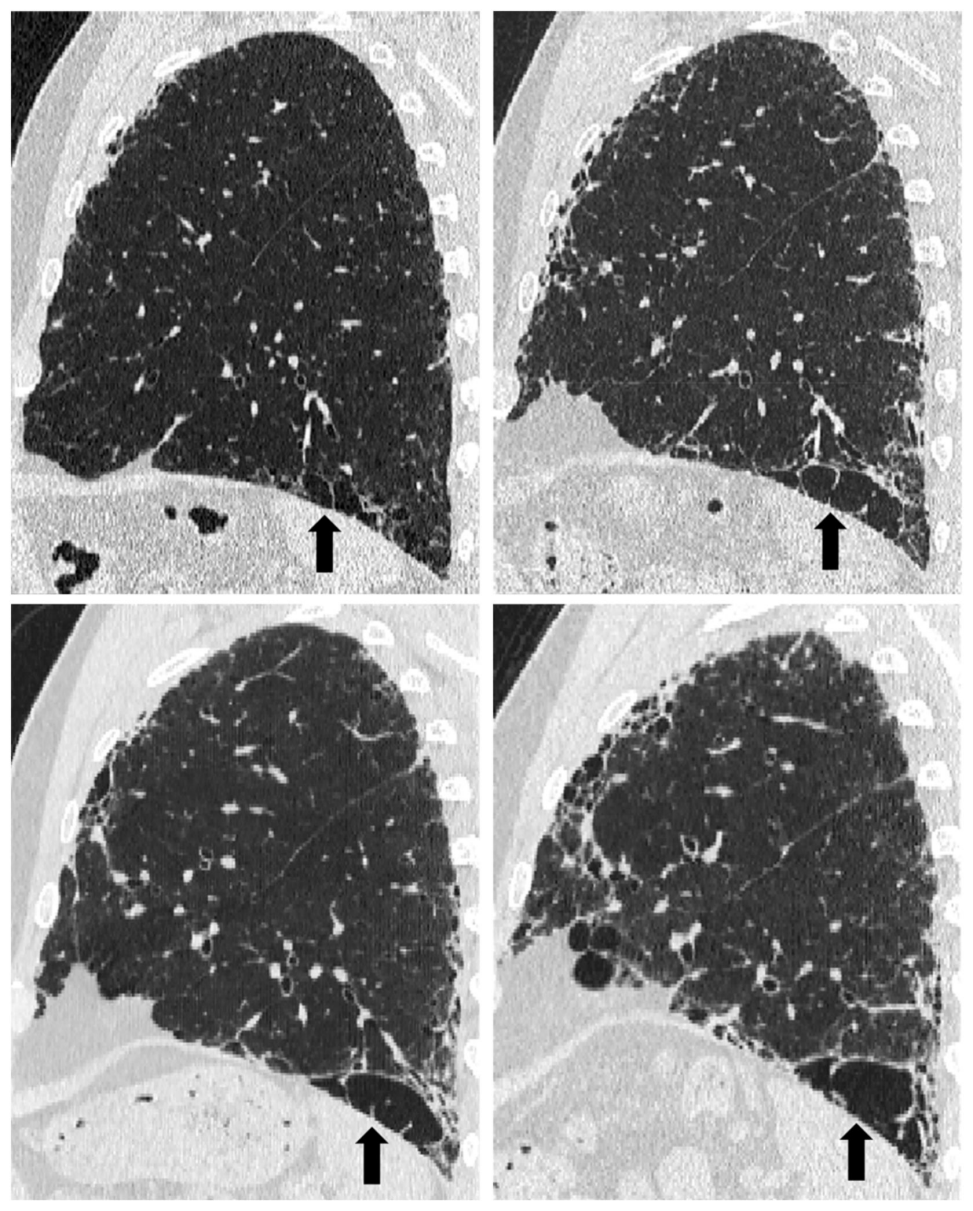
Sagittal CT images of the lungs performed over the course of 4 years in a 66-year-old male ex-smoker diagnosed with idiopathic pulmonary fibrosis and emphysema (combined pulmonary fibrosis and emphysema, thick-walled large cysts pattern). In the top left image low-attenuation lesions without clearly visible walls in keeping with emphysema (arrow) are visible adjacent to the diaphragm and lie within fibrotic regions of lung. Over the next 4 years, as the fibrosis matures, the low-attenuation lesions coalesce (arrow) and enlarge in size forming a thick-walled cystic lesion.
